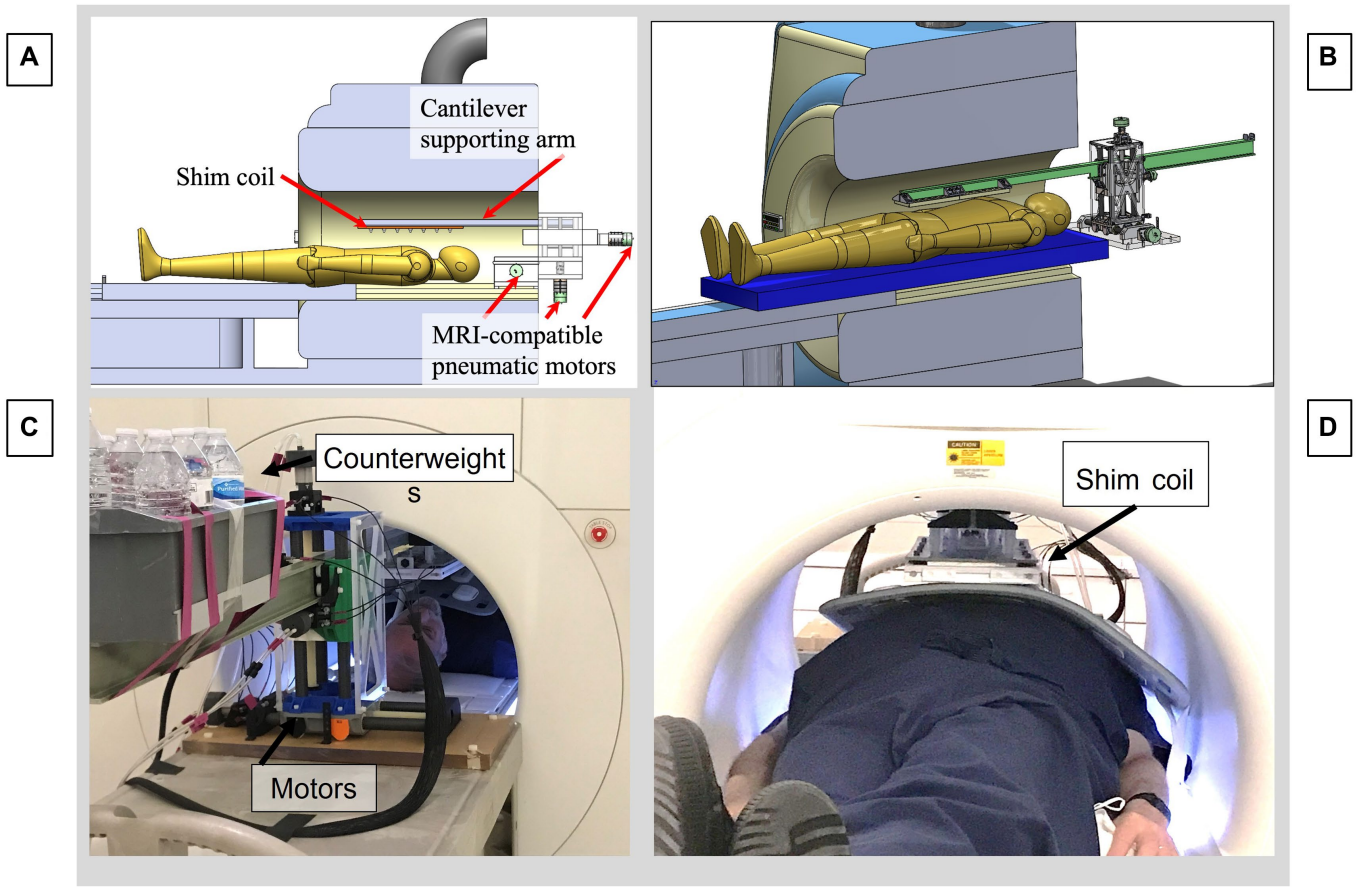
Motorized cantilevered system to remotely position the shim coil in the MRI. (A) CAD model of the entire system. (B) CAD focusing on cantilevered arm and motors. (C) View from rear of MRI bore, showing pneumatic motors and back end of cantilevered beam, with counterweights balancing the moment arm produced by the shim coil. (D) View from front of bore, showing subject inside the bore with a cardiac coil above the abdomen with the shim coil above, suspended from the distal end of the beam.
